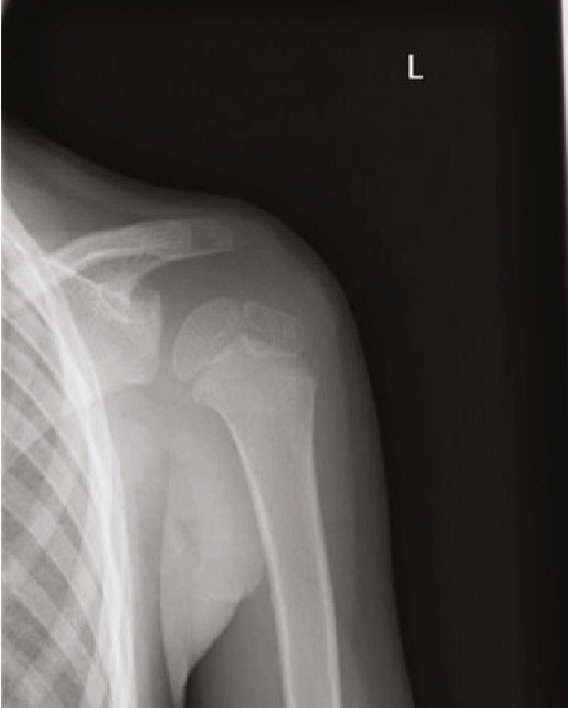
Radiograph at final follow-up.
